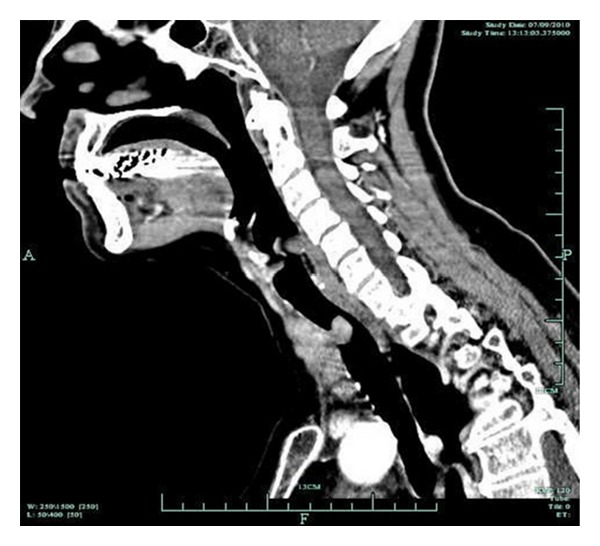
CT scan of the neck and mediastinum which revealed a mass originating from the thyroid gland, invading and severely obstructing the lumen of the trachea.
